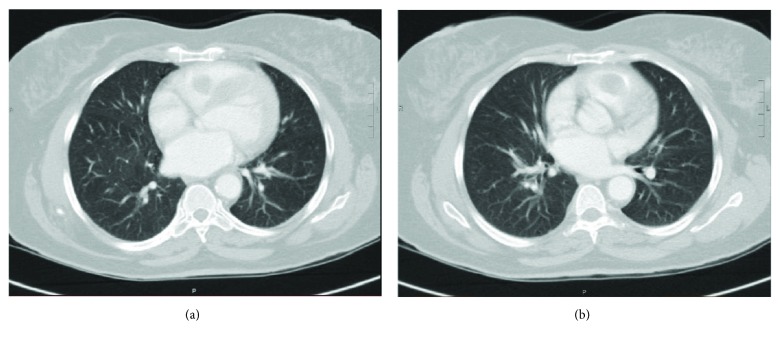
Chest CT scan with IV contrast showing a filling defect within the contrast-enhanced right ventricle which was initially thought to be a large thrombus extending to the right ventricular outflow tract.
